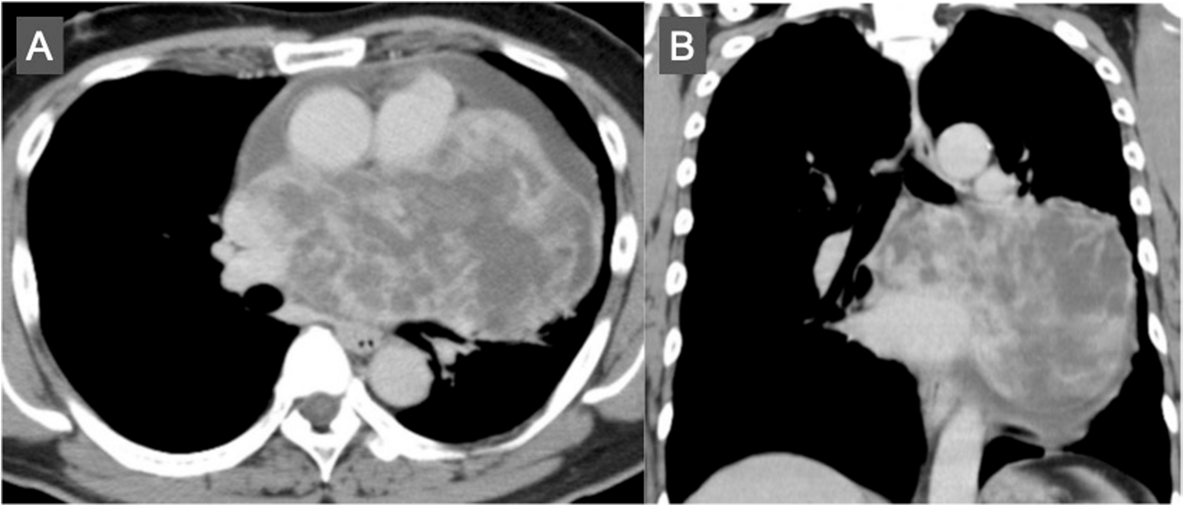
Preoperative computed tomography. A huge mass situates inside the pericardial cavity and compresses the heart. The content of the mass looks solid and heterogeneous with contrast medium
